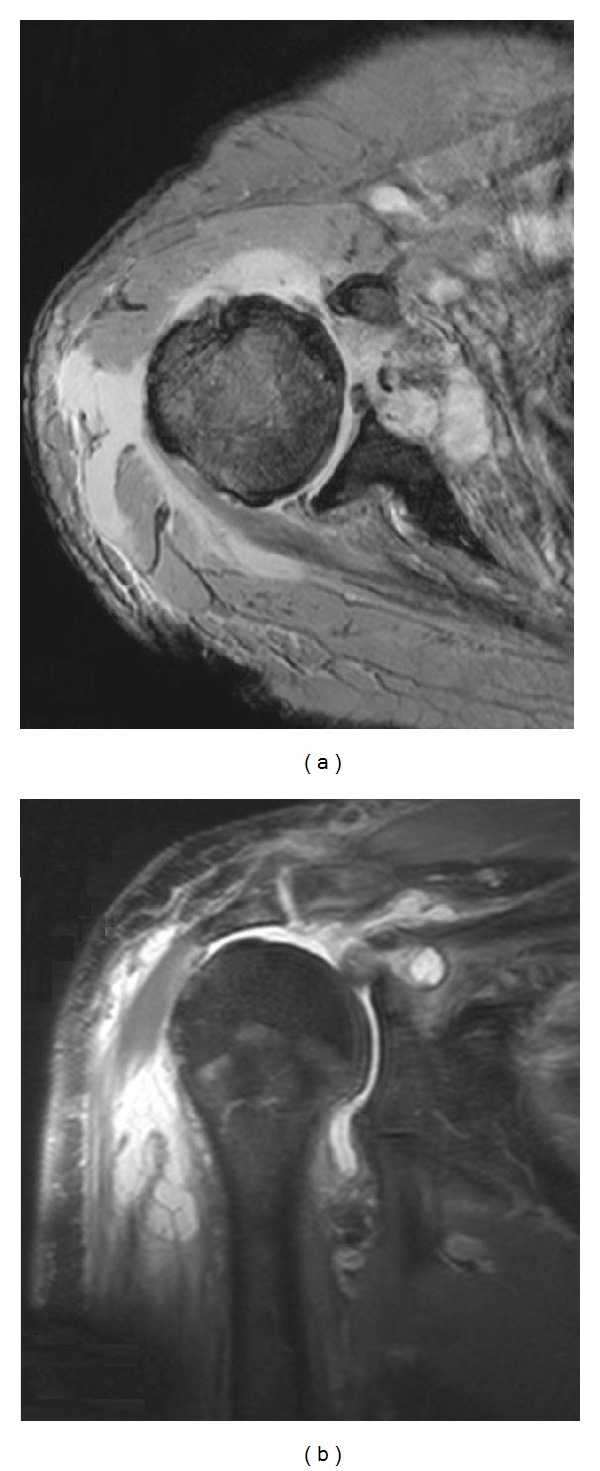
T2 Weighted MRI Images of the right shoulder taken at 22 hours after presentation, showing early osteomyelitis, bone marrow oedema, bursal collection, and high signal consistent with septic arthritis.
